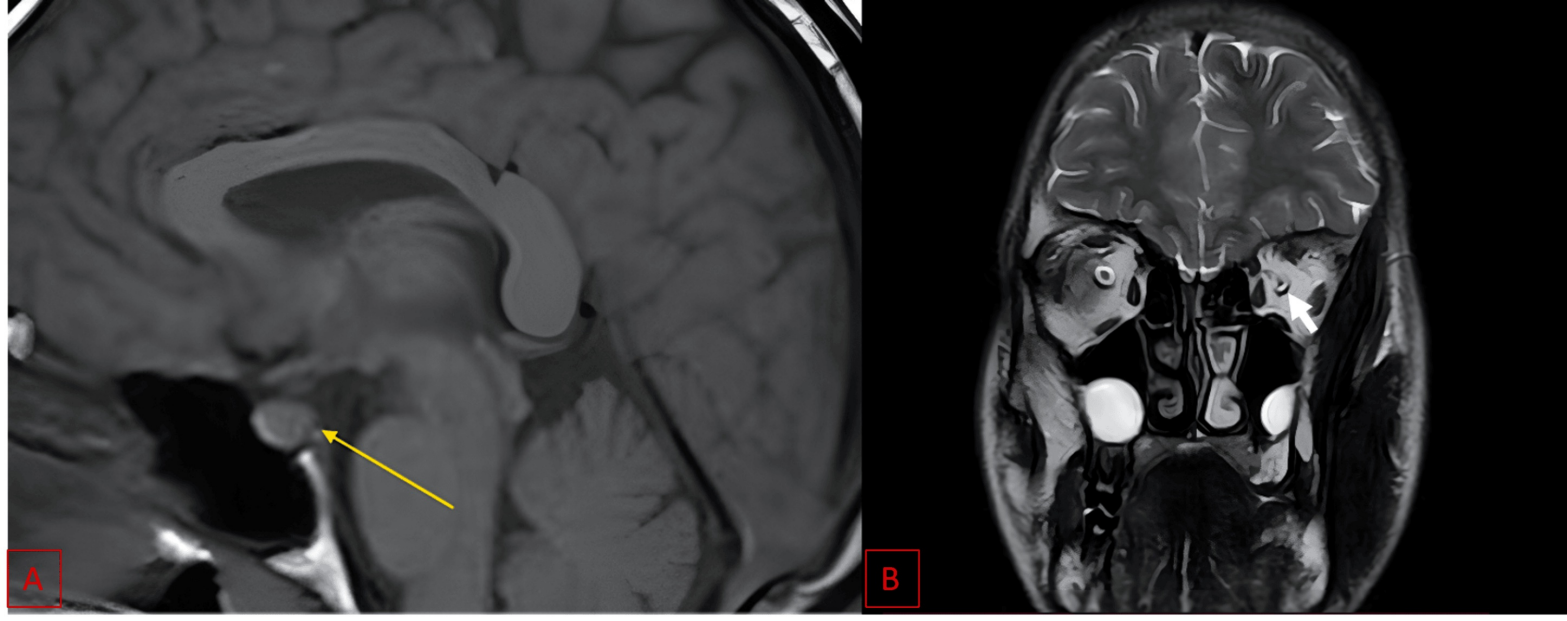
Saggital and Coronal MRI images. A: Sagittal T1-weighted brain MRI shows absence of the normal hyperintense posterior pituitary bright spot (yellow arrow), consistent with posterior pituitary agenesis. B: Coronal T2-weighted images demonstrate bilateral optic nerve atrophy, more marked on the left (white arrow), with no evidence of compressive or infiltrative lesions.
